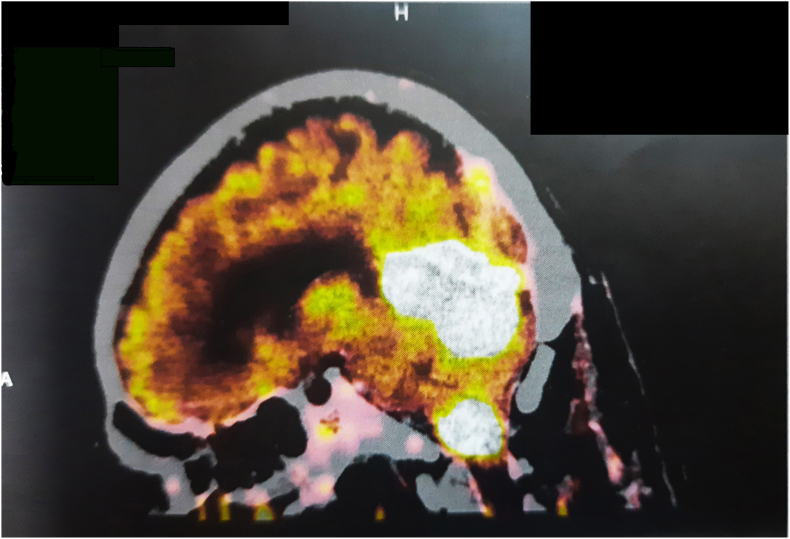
18F-FDG PET-CECT brain shows two discrete FDG avid enhancing masses.
